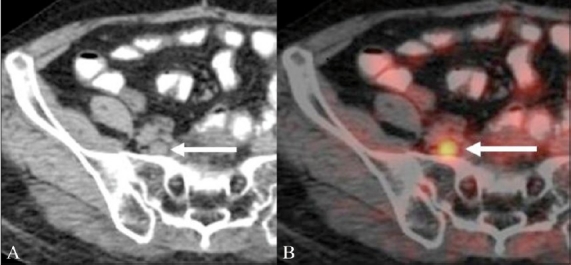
A 62-year-old man treated for CRC was imaged with FDG PET / CT during routine surveillance. An axial unenhanced CT scan (A) reveals a sub-centimeter-sized, round, right common iliac node (arrow) that shows increased FDG uptake (arrow) on the fusion PET / CT image (B). USG-guided fine needle biopsy confirmed nodal recurrence
